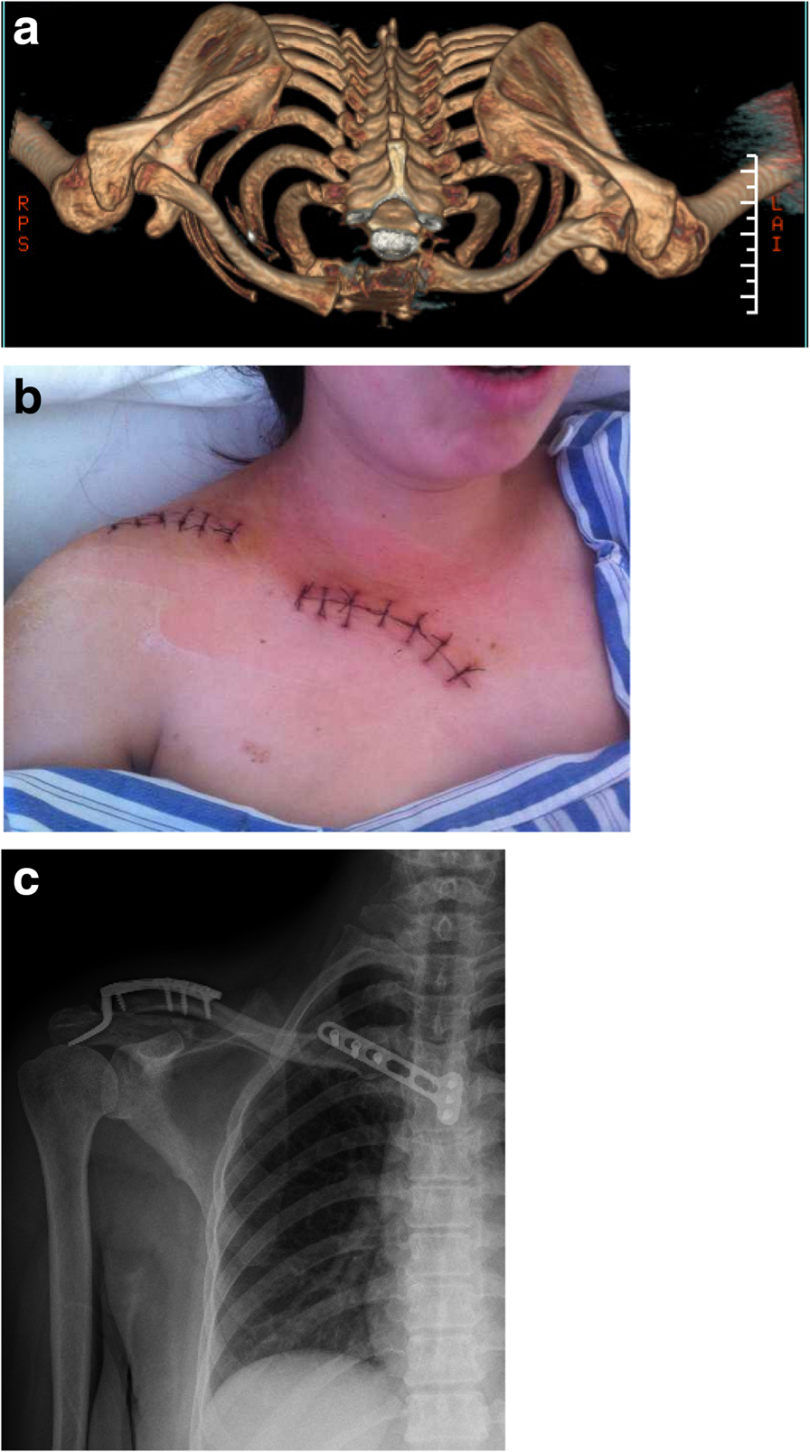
Preoperative a Initial transverse and sagittal computed tomography scans of both sides of the shoulder girdle showing a posterior acromioclavicular joint dislocation (Rockwood type IV) and an anterior sternoclavicular joint dislocation, b The final operative incision and c postoperative x-ray
